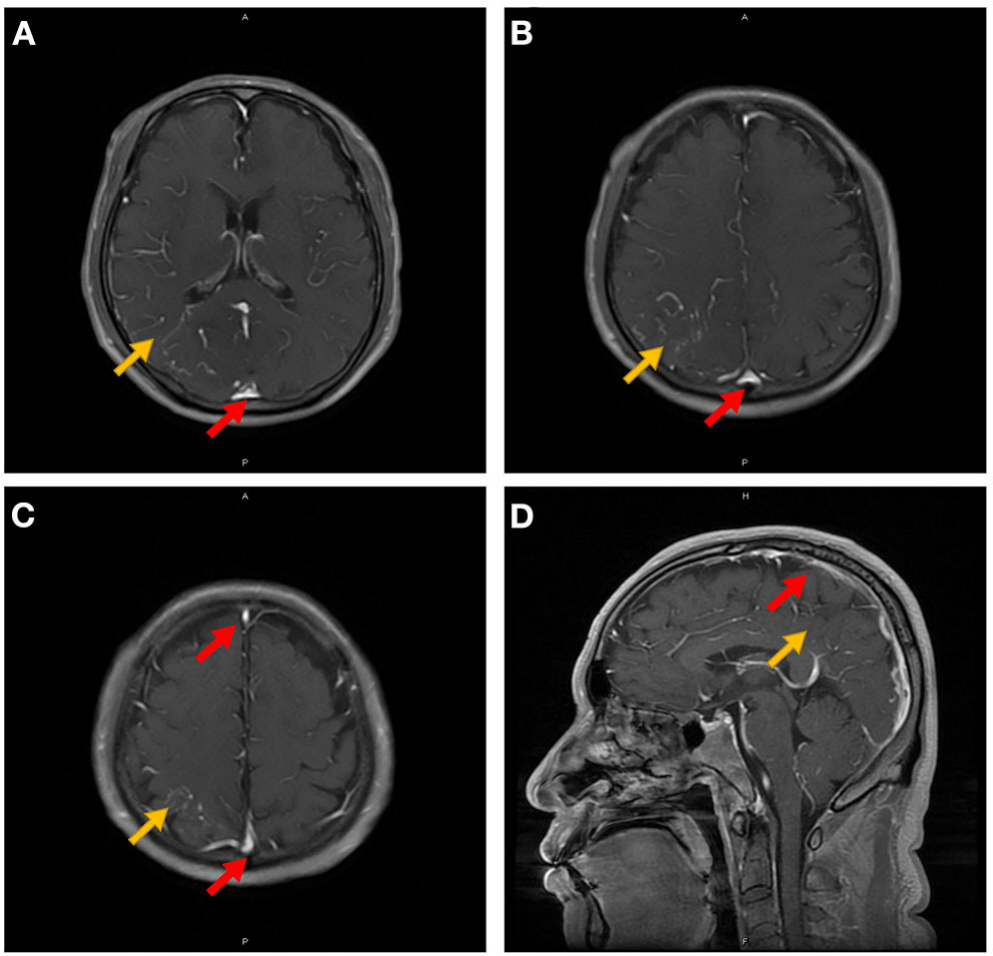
Contrast-enhanced MRI of the brain in Case 5. The orange arrow indicates leptomeningeal or medullary vascular enhancement in the right parietal-occipital lobe, and the red arrow indicates the simultaneous venous sinus enhancement (A–D).
